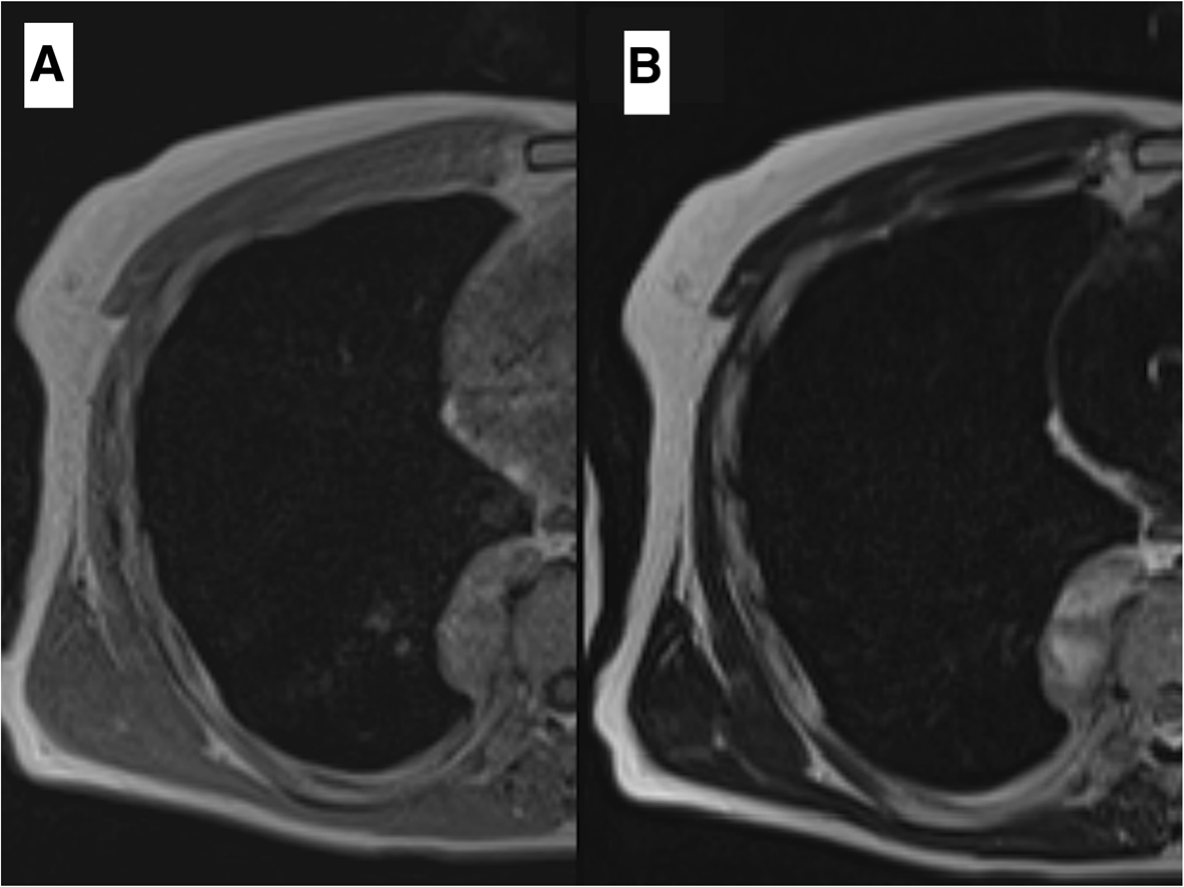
Chest MRI revealed that the signal intensity of the tumor was low in the muscles in the T1 weighted image (a) and disproportionately high in the T2 weighted image (b). The tumor invaded neither the intervertebral foramen nor the vertebral body
